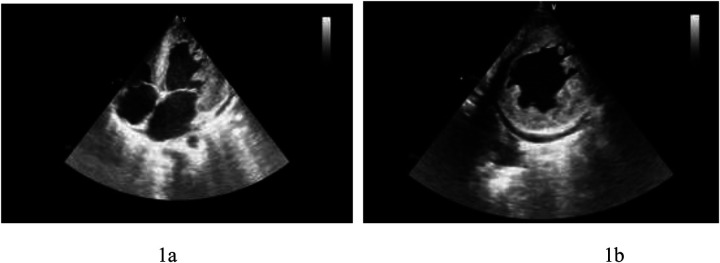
(a) Left ventricular apical four-chamber view showing left ventricular thickening and hypertrabeculation. (b) Left ventricular short-axis view presenting left ventricular thickening and hypertrabeculation.
